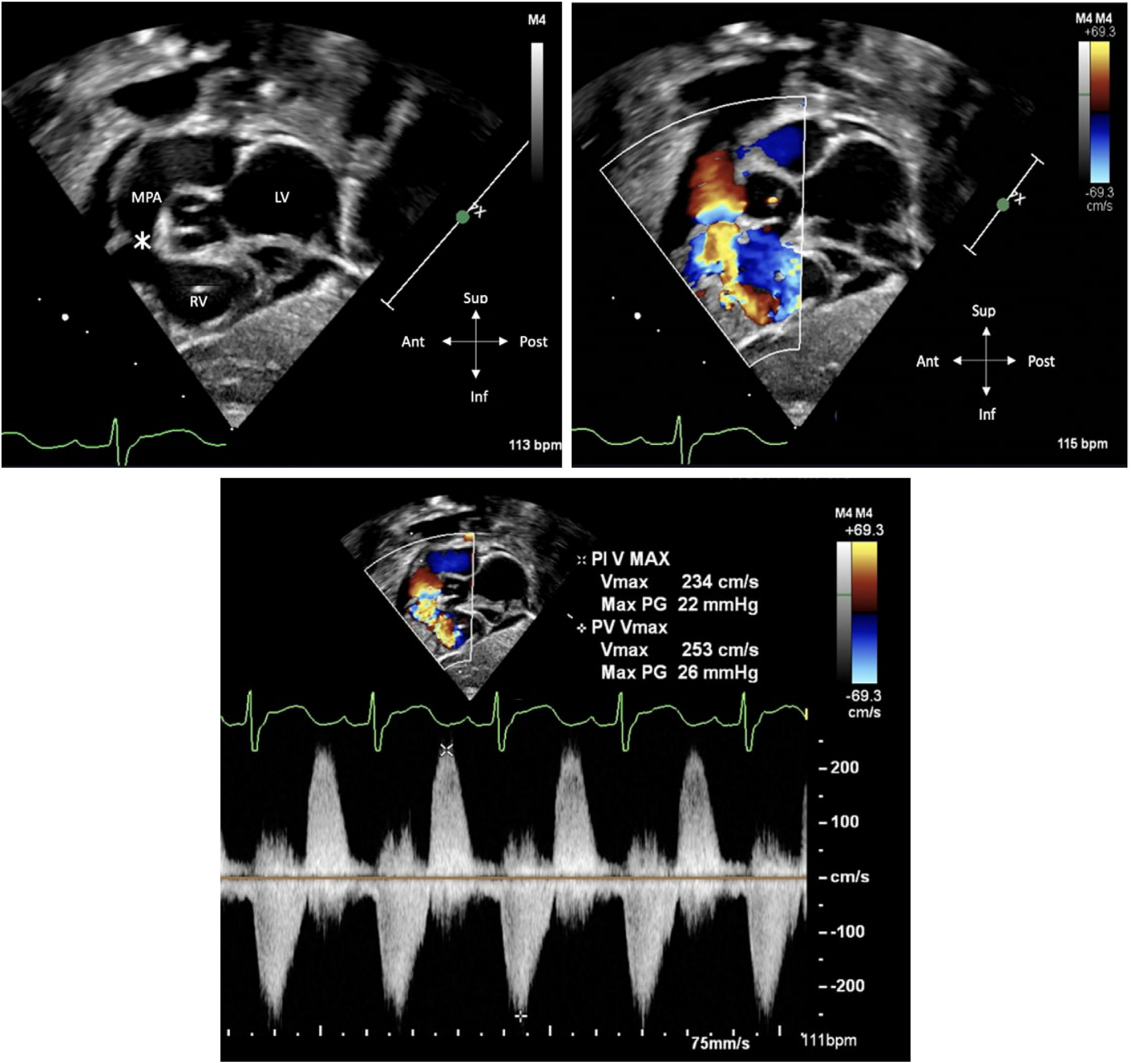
Postnatal two-dimensional transthoracic echocardiogram, subcostal sagittal view, without (*top left*) and with (*top right*) color-flow Doppler, diastolic phase, demonstrates a hypoplastic and hypertrophied RV and no evidence of PV leaflets (*asterisk*) with flow reversal in the MPA due to absence of PV leaflets. Continuous-wave Doppler demonstrates systolic antegrade and diastolic retrograde flow due to absence of valve apparatus (*bottom*).
